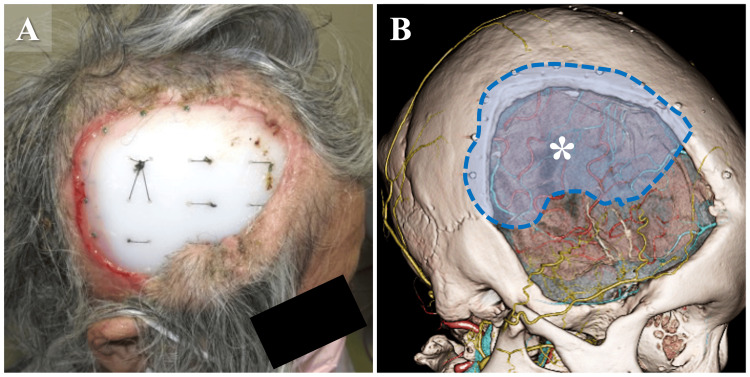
Preoperative findings on admission. (A) Preoperative appearance on admission showing a large scalp defect with exposure of the synthetic cranial implant. (B) Three-dimensional CT angiography demonstrating the scalp defect (white asterisk) and the superficial temporal and occipital arteries highlighted in yellow.
